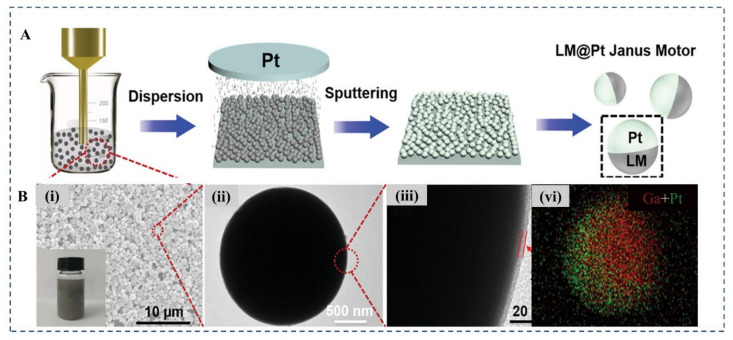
Two-steps fabrication methods for MLMTs. (A) Fabrication of the LM-Pt Janus particles via sputtering Pt onto LMNPs produced by ultrasonication [6]. (B) (i) SEM image of the LM spheres fabricated by ultrasonic dispersion. (ii) The symmetric LM sphere fabricated by ultrasonication is shown by the TEM image. (iii) A thin Ga_2_O_3_ shell on the LM sphere. (vi) The LM-Pt the distribution of the asymmetric elements after spurting is shown by EDS elemental mapping. [6].
